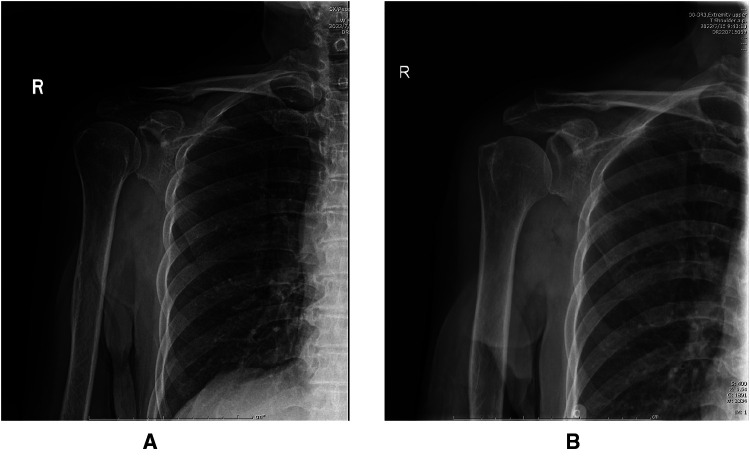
(A) Preoperative x-ray of the patient with supraspinatus calcific tendonitis calcifications. (B) 1 week after surgery, calcifications cannot be detected on x-rays.
